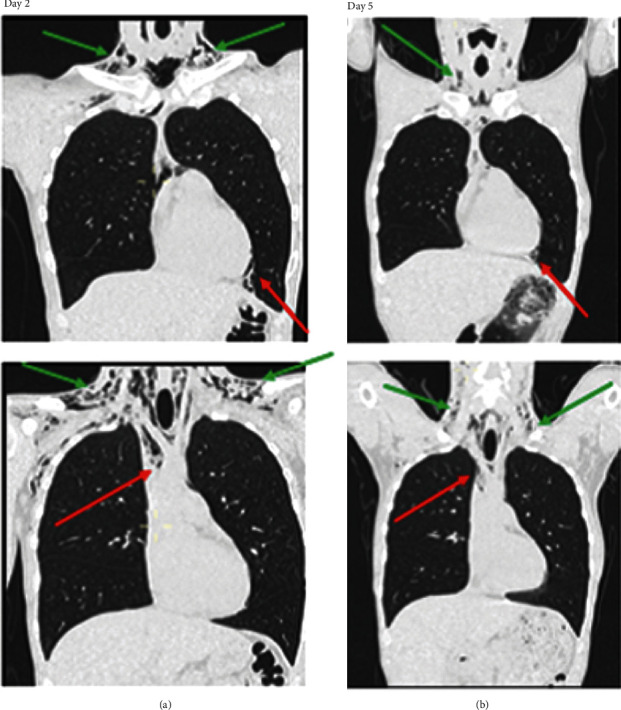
Cervicothoracic frontal slices obtained during the computed tomography scan. Subcutaneous supraclavicular soft tissue emphysema (green arrow) and pneumomediastinum (red arrow) from 2nd to the 5th day after extraction are reduced. (a) Cervicothoracic tomography scan, frontal slices, 2nd day after wisdom tooth extraction. (b) Cervicothoracic tomography scan, frontal slices, 5nd day after wisdom tooth extraction.
